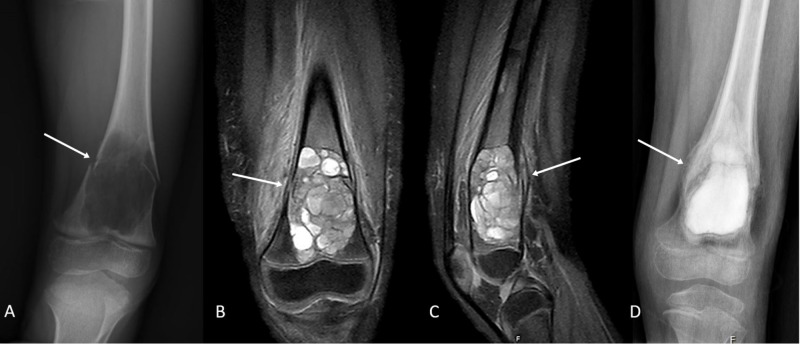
(A) Plain anteroposterior radiographic film demonstrating a left femoral distal metaphyseal expansile lytic lesion with a fallen leaf type fracture. (B,C) Coronal and sagittal magnetic resonance (MR) images demonstrating expansile lesion of the distal metaphysis of the femur. No underlying soft tissue elements are noted, suggesting the lesion is most likely a primary aneurysmal bone cyst. (D) Plain anteroposterior radiographic film demonstrating callus formation and postop changes at six weeks of follow-up.
